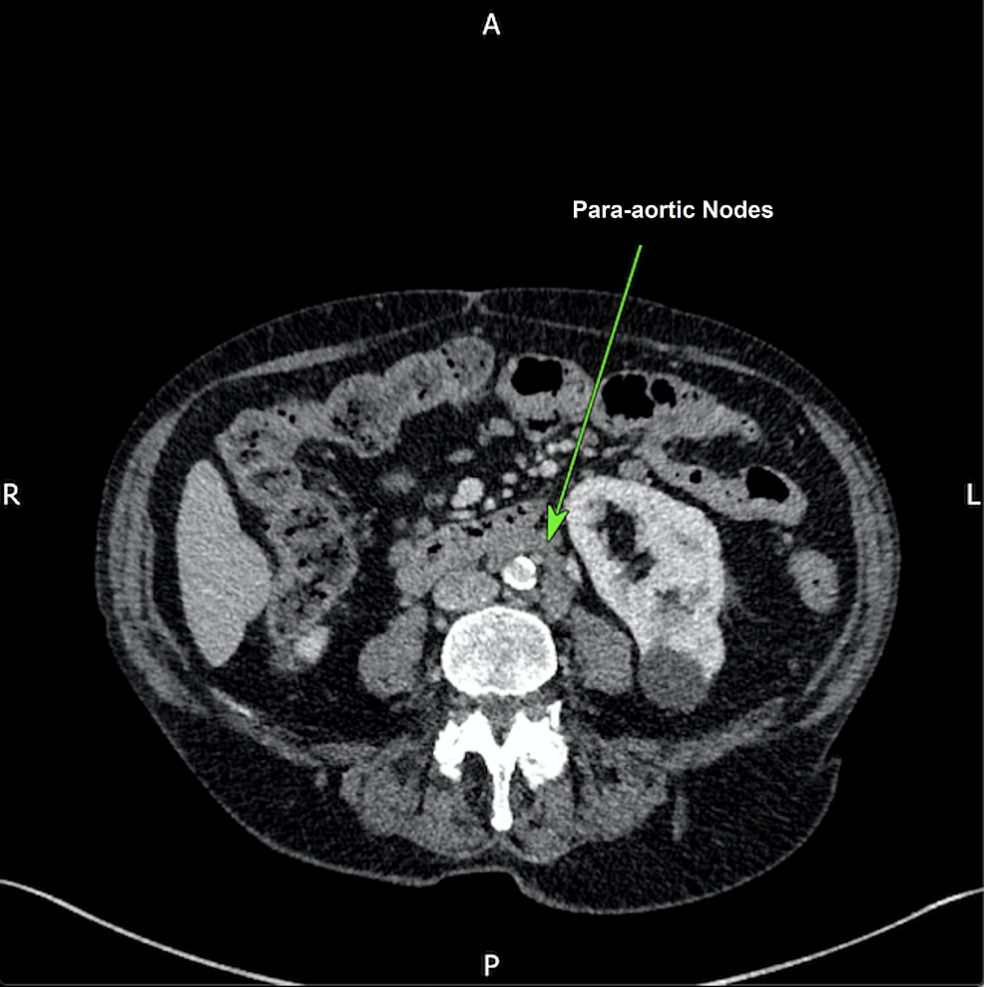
Computed tomography (CT) of the abdomen displaying enlarged para-aortic lymph nodes (green arrow).
